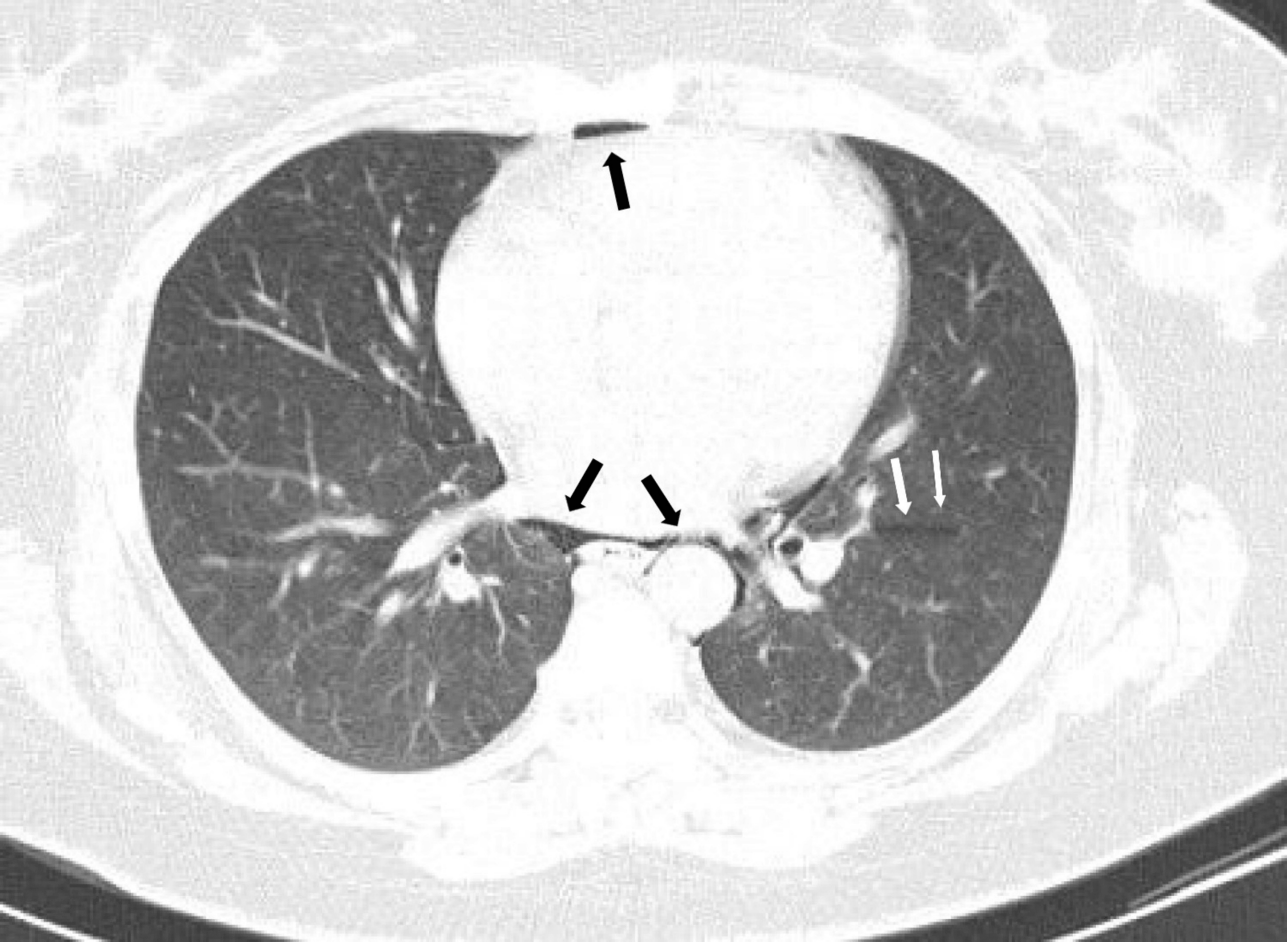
Chest CT scan (transverse section) showing pneumomediastinum due to the Macklin effect The chest CT scan confirmed pneumomediastinum (black arrows) secondary to the Macklin effect, associated with interstitial emphysema dissecting along the peribronchovascular sheaths (white arrows).
